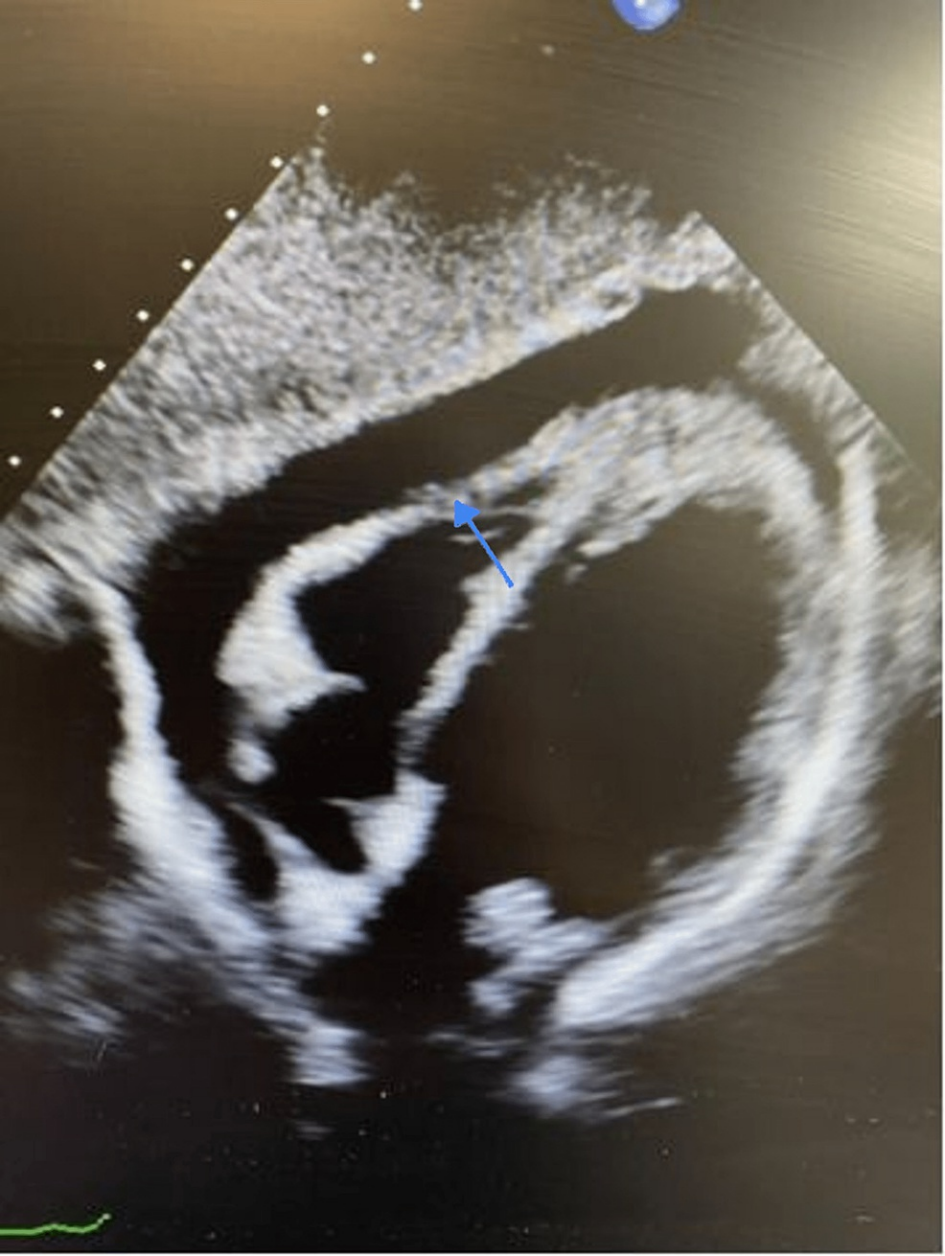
Severe PE on apical four-chamber echocardiography
